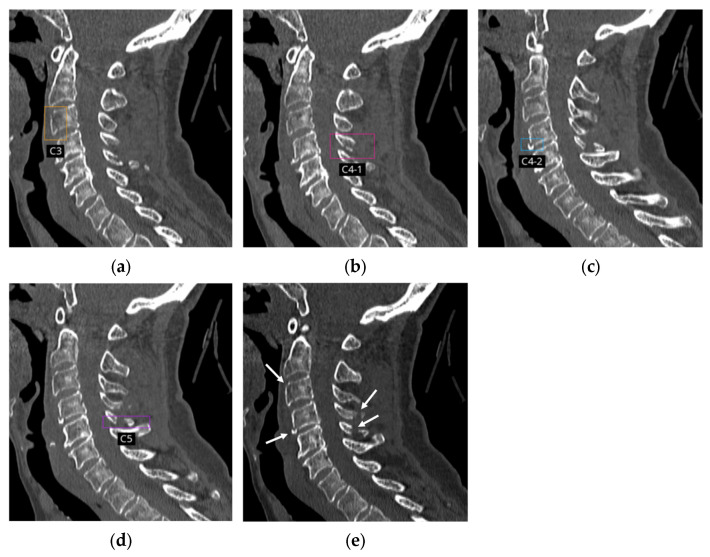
Non-enhanced CT images in a 73-year-old female with four acute fractures of the C3 (vertebral body), C4 (vertebral body and spinous process), and C5 (spinous process), as agreed upon by the first two radiologists. (a–d) The AI application correctly localized all fractures using bounding boxes and accurately labeled the corresponding spinal levels. Because two fractures were present within C4, the lesions are labeled as C4-1 and C4-2. (e) Corresponding sagittal CT image without bounding boxes shows the four fractures (arrows).
